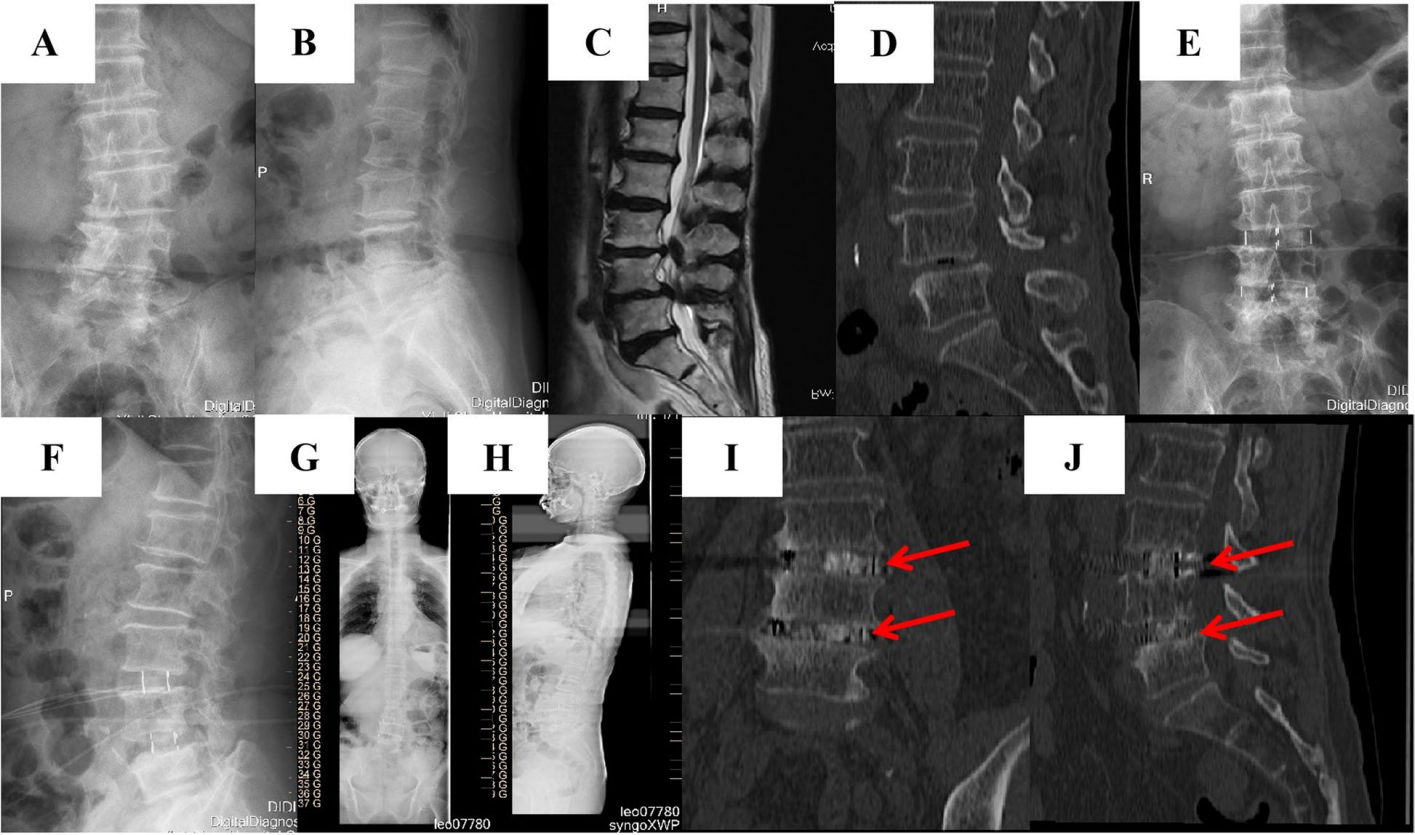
A typical case of a 66-year-old patient presented with mechanical back pain. The preoperative radiograph indicated degenerative scoliosis (A-B), preoperative MRI and CT showed lumbar stenosis of L3/4 and L4/5 (C-D). Postoperative X-ray (E-F) and standing Full length film of spine (G-H) after 1 year showed that the coronal and sagittal balance had been corrected. The 3D-CT scan indicated bony fusion of L3/4 and L4/5 in terms of coronal (I) and sagittal (J) reconstruction
